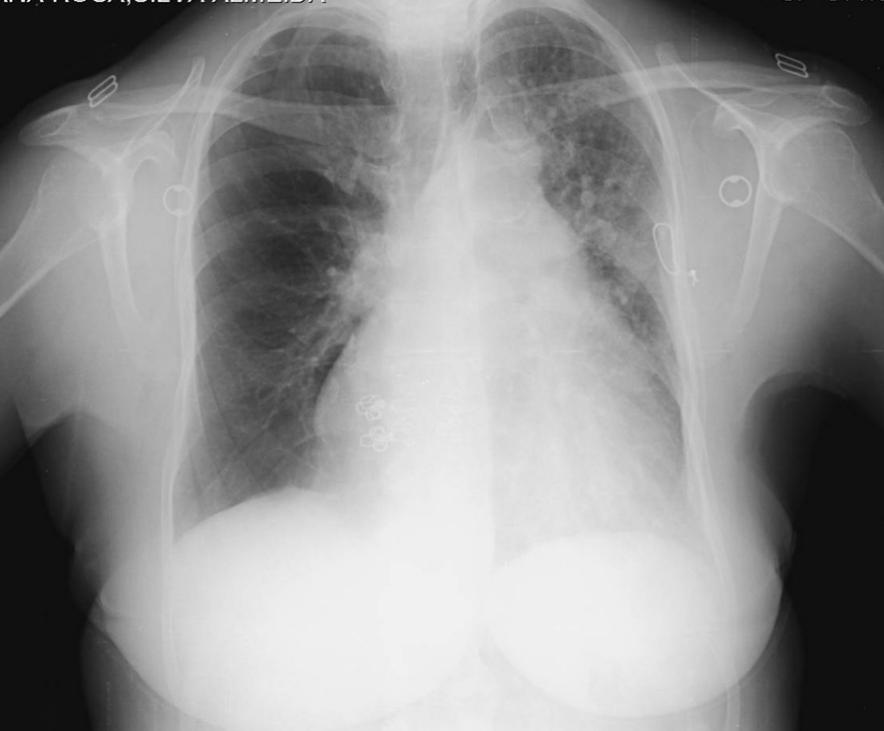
Chest X-ray on admission to the emergency department.
